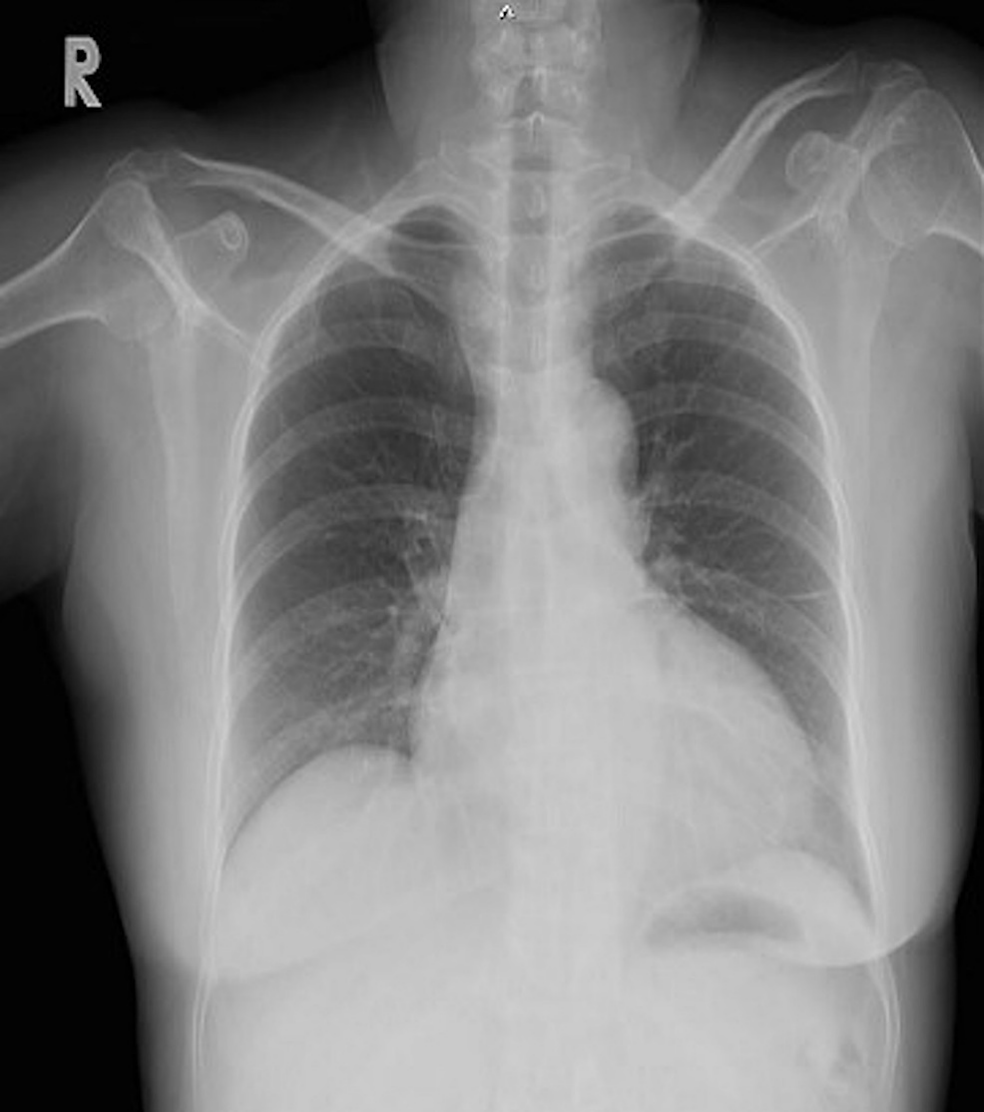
Chest x-ray (posteroanterior view)
